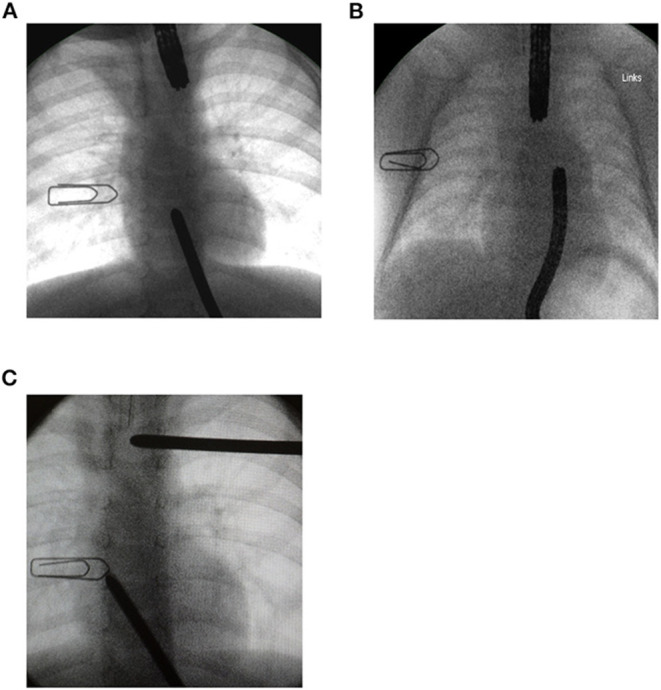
Radiographic gap assessment at 3 months of age. A 1 cm wide paperclip was placed on the patient's chest as a reference to measure gap length. (A) A flexible endoscope was placed in the upper pouch while a Hegar dilator marked the lower pouch. (B) Flexible endoscopes placed in each pouch. (C) One Hegar dilator placed at the site of spit fistula while the tip of the second dilator marked the upper end of the lower pouch.
